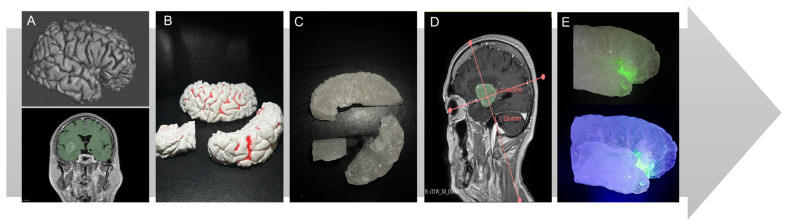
(A) The segmented right hemisphere in Meshmixer by Autodesk (San Francisco, CA, USA) and segmentation of the brain’s right hemisphere. (B) The 3D-printed sections of the Sylvian fissure. (C) Sylvian fissure sections cast in candle gel. (D) The insular glioma in MRI scan after Berger–Sanai. (E) The assembled Sylvian fissure sections with the integrated fluorescent glioma without blue light and with blue light.
